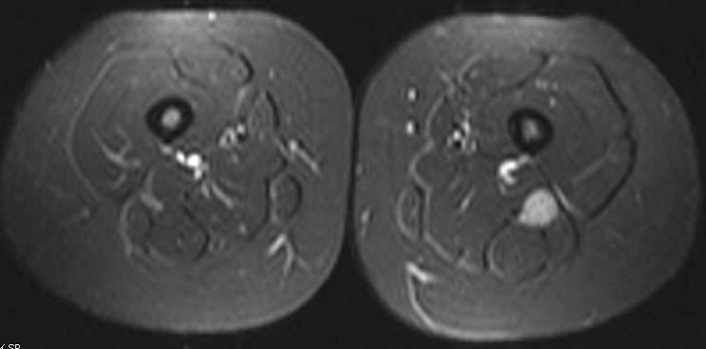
T2-weighted image showing enlargement of the sciatic nerve when compared to the other side.
